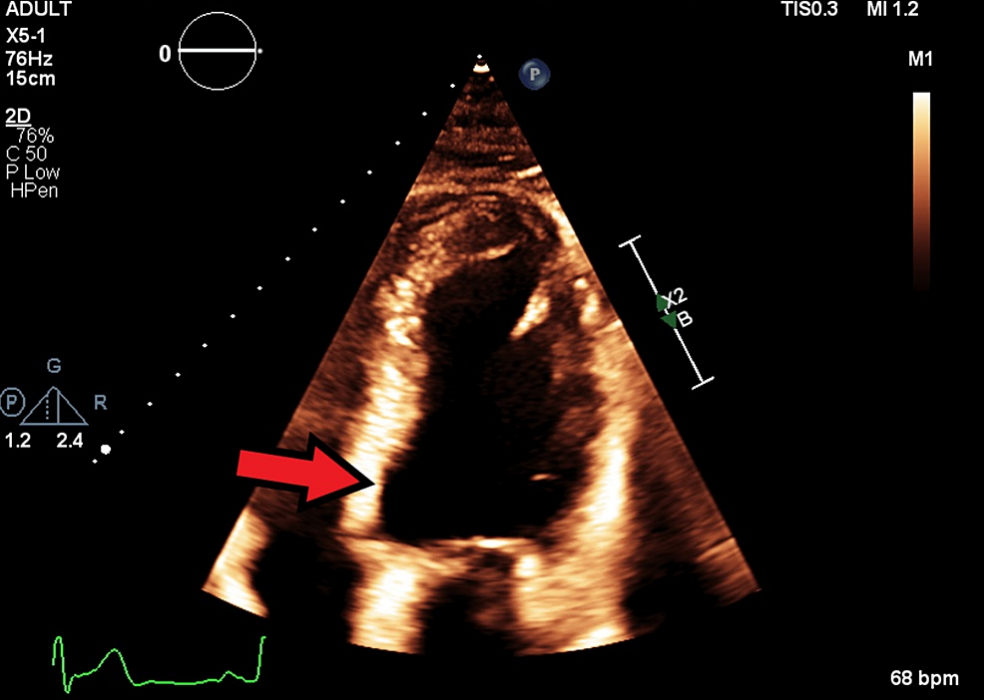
Image from baseline echocardiogram prior to treatment revealed normal ejection fraction (EF) of 61%, normal ventricular cavity size and wall thickness, and no valvular or wall motion abnormalities.
